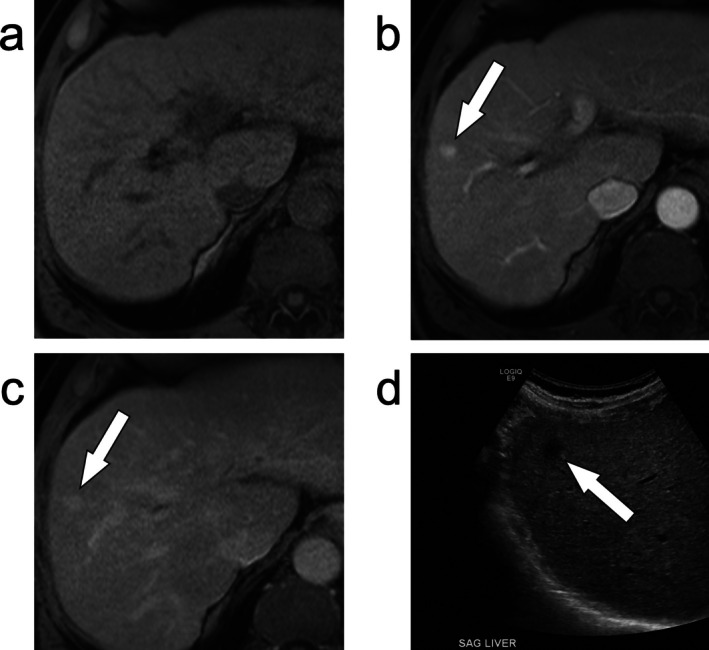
An axial T_1_‐weighted fat‐suppressed image of the liver (a) does not demonstrate any liver observations. Following the administration of intravenous gadolinium, a 1 cm focal observation demonstrated enhancement that persisted on the portal venous phase (b, arrow) and delayed phase (c, arrow). This fulfilled LR‐3 criteria (intermediate probability of malignancy) based on the major feature table. However, a prior ultrasound showed a corresponding lesion (d, arrow), which is an ancillary feature favoring malignancy in general, not HCC in particular. This observation was categorized as LR‐4 (probably HCC).
